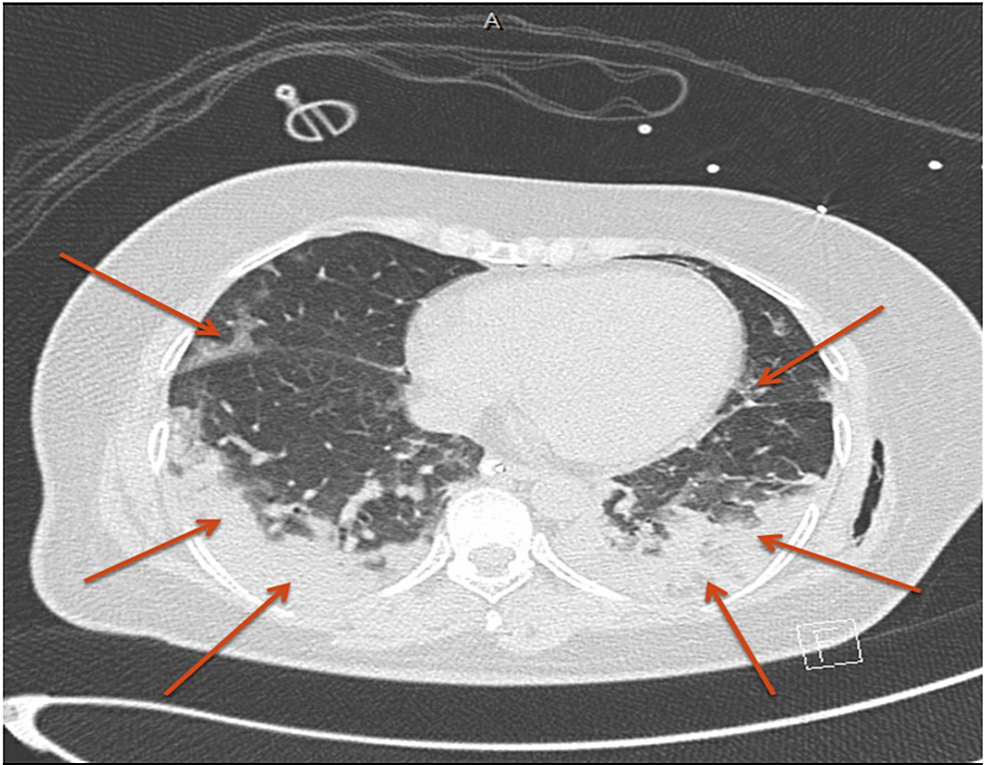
CT thorax showing bilateral basal large consolidations associated with multifocal peripheral ground glass opacities, typical for COVID-19 pneumonia.
